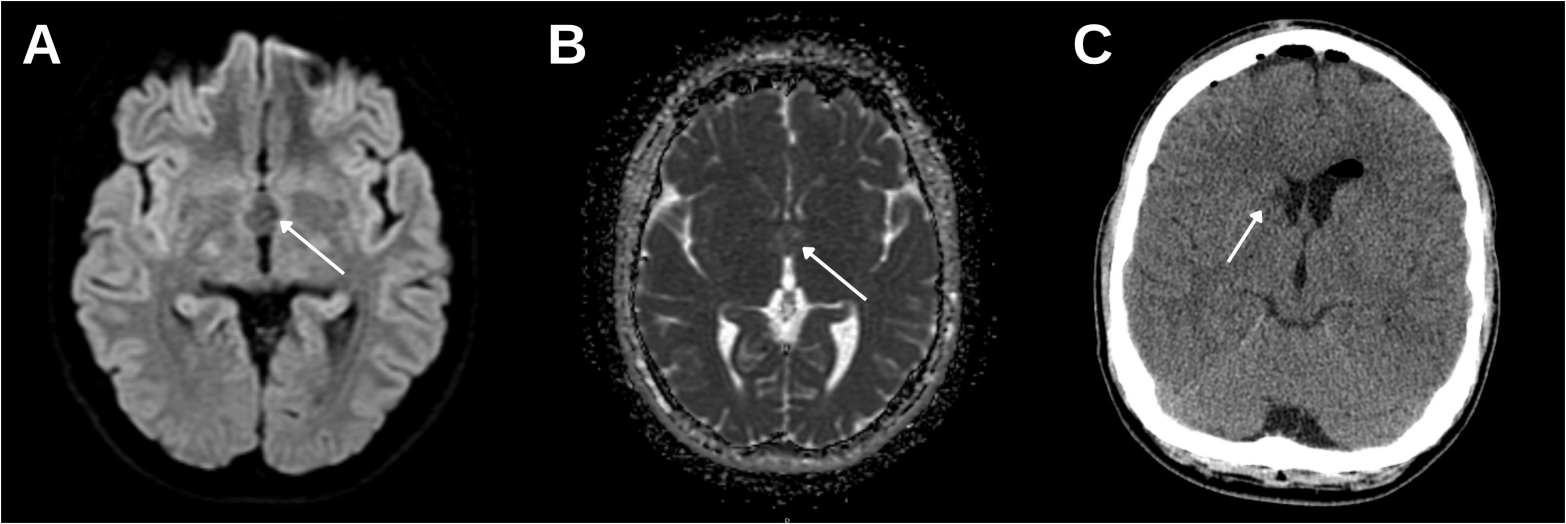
MR diffusion imaging using 1.5 T and postoperative CT. (A) Axial DWI shows no evidence of restricted diffusion. (B) Corresponding ADC map shows no reduction in ADC value; no imaging features suspicious for malignant transformation are seen. (C) Postoperative non-contrast head CT shows interval removal of the intraventricular mass with expected changes around the foramen of Monro; minimal postoperative edema is present at the caudate head (arrow), with no acute hemorrhage or ventriculomegaly.
